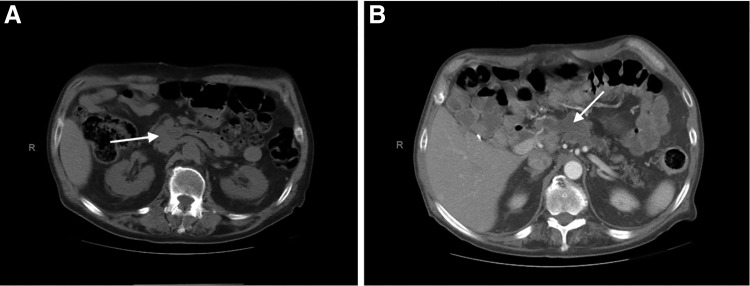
CT scans, with *arrows* showing cystic mass (A) and diffuse ductal dilation (B).
